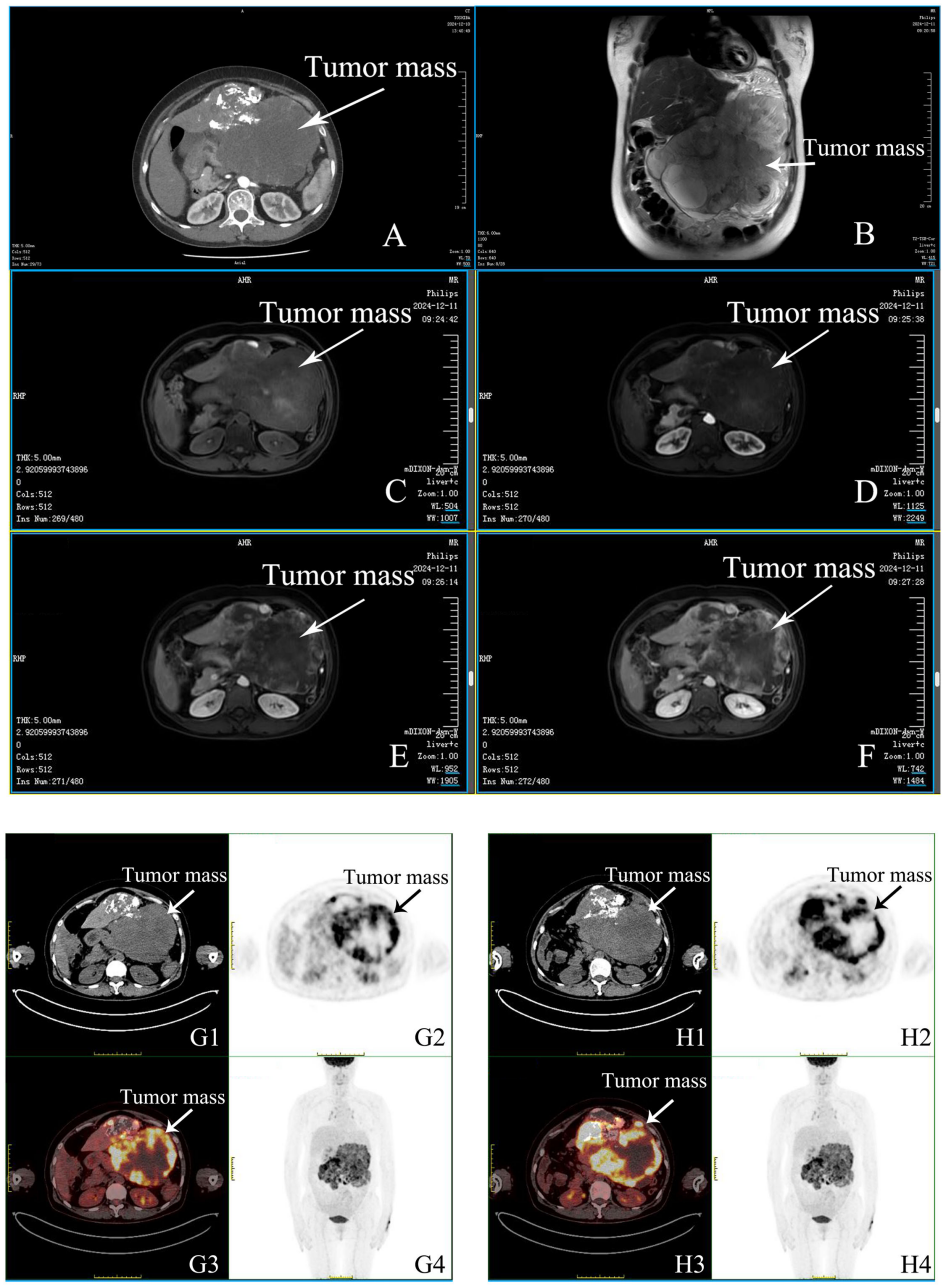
Imaging findings of post-treatment hepatocellular carcinoma with associated complications. (A) Contrast-enhanced CT (CTA, CTV) demonstrates post-interventional changes in the liver. The left hepatic lobe shows a large heterogeneous, hypodense mass extending into the abdominal cavity (22.4 × 12.6 cm). The mass contains multiple hypodense areas with iodized oil deposition. Arterial phase imaging shows peripheral enhancement, while portal and delayed phases show hypodensity relative to the surrounding liver parenchyma. Additional round, non-enhancing foci are observed within the liver. Normal biliary structures are noted. Splenic vein stenosis and possible right adnexal mass with pelvic effusion are evident. (B) Axial T2-weighted MRI reveals a large mixed-signal mass in the left hepatic lobe (22.9 × 13.7 cm) protruding into the abdominal cavity. The lesion shows multiple long T1 and T2 signal areas suggestive of necrosis or fluid. (C–F) Axial mDIXON MRI sequences show the liver lesion with heterogeneous signal intensities. Arterial phase enhancement highlights active tumor areas, while non-enhancing regions correspond to necrotic or cystic components. Associated findings include a hepatic cyst and adjacent vascular and intestinal displacement. (G1–H1) Axial CT scans of the abdomen show a large exophytic mass in the left hepatic lobe (22.1 × 13.9 cm), containing mixed densities with iodized oil deposition and necrotic areas. Mildly enlarged lymph nodes are observed in the mediastinum (SUVmax 5.5). (G2–H2) PET scans display regions of increased metabolic activity within the hepatic lesion, with a maximum SUV of 17.4, indicating residual tumor activity. (G3–H3) Fused PET/CT images highlight the precise localization of metabolic activity within the hepatic mass and mediastinal lymph nodes, combining anatomical and functional data. (G4–H4) The whole-body PET scan shows no evidence of distant metastasis. Background uptake is normal in the brain, thyroid, and other major organs. Moderate pelvic effusion and right adnexal mass (3.8 × 2.1 cm) are seen, with low metabolic activity. Additional mild uptake (SUVmax 2.3) is noted in a small nodule in the left upper lobe of the lung, suggesting an inflammatory process.
